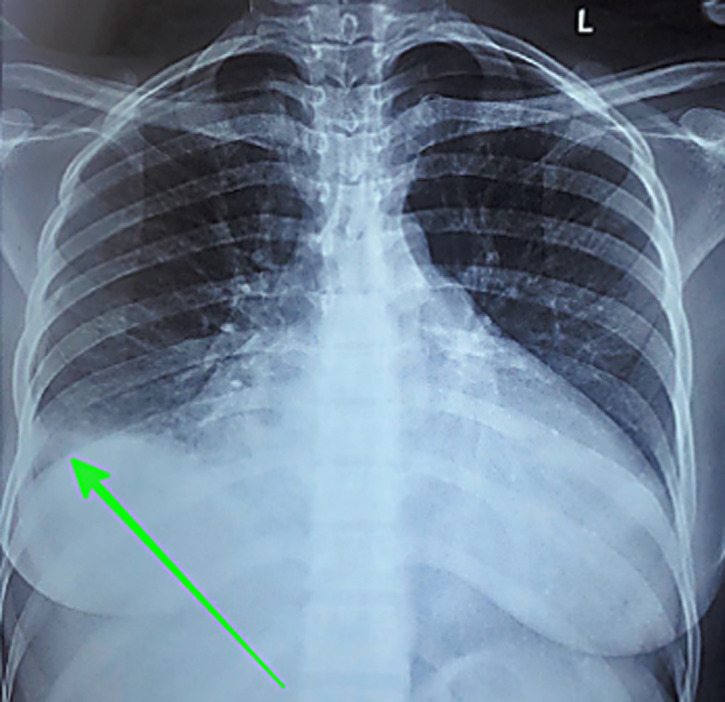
chest X-ray showing a right pleural effusion (green arrow)
